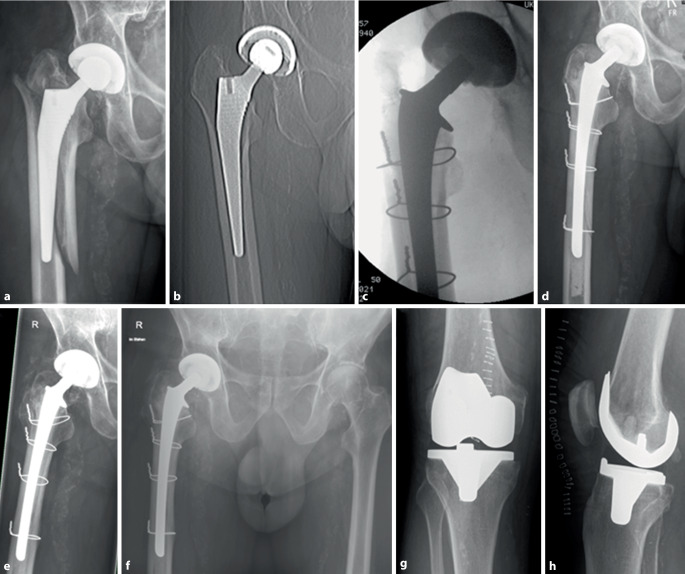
a 81 Jahre, männlich. Periprothetische Femurfraktur (PPF) Vancouver Typ B2 nach Sturzereignis. b CT-Topogramm einer früheren Untersuchung des Beckens, welches dem prätraumatischen Vergleich dient. c Intraoperatives Bildwandlerbild nach Osteosynthese (1,5 mm Drahtcerclage) und Schaftwechsel. d Postoperative Kontrolle nach Revisionsoperation mit anatomischer Frakturreposition sowie zementiertem Schaftwechsel. Postoperativ erfolgte unmittelbar die schmerzadaptierte Vollbelastung. e/f Röntgenaufnahme Hüftgelenk axial und Beckenübersicht. In der 1‑Jahres-Kontrolle zeigt sich eine konsolidierte Fraktur. g/h Röntgen Kniegelenk a.p. und seitlich. Bei guter postoperativer Mobilität und ipsilateral fortgeschrittener Gonarthrose erfolgte 1,5 Jahre nach der PPF die Implantation eines bikondylären Oberflächenersatzes
